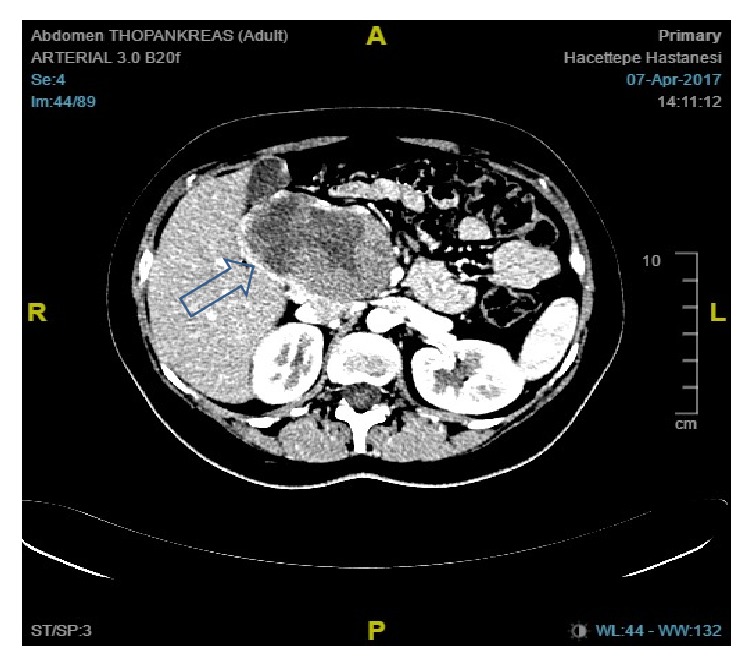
Contrast-enhanced CT of the abdomen showing the mass at the pancreatic head (arrow).
